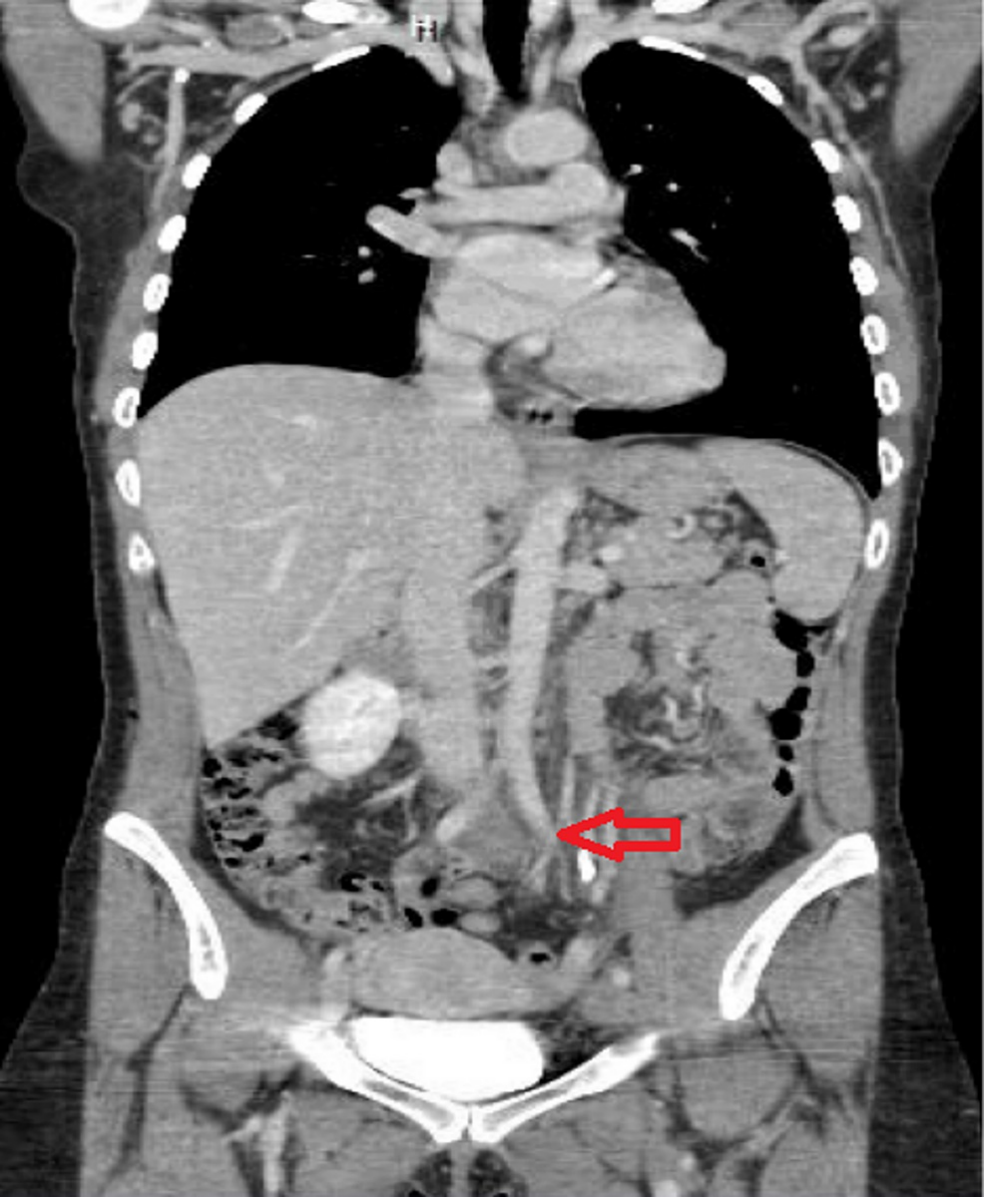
Computed tomography (CT) chest, abdomen, and pelvis with intravenous contrast The left common iliac vein, left external iliac vein, and sections of the left internal iliac vein were all found to be thrombosed (arrow).
